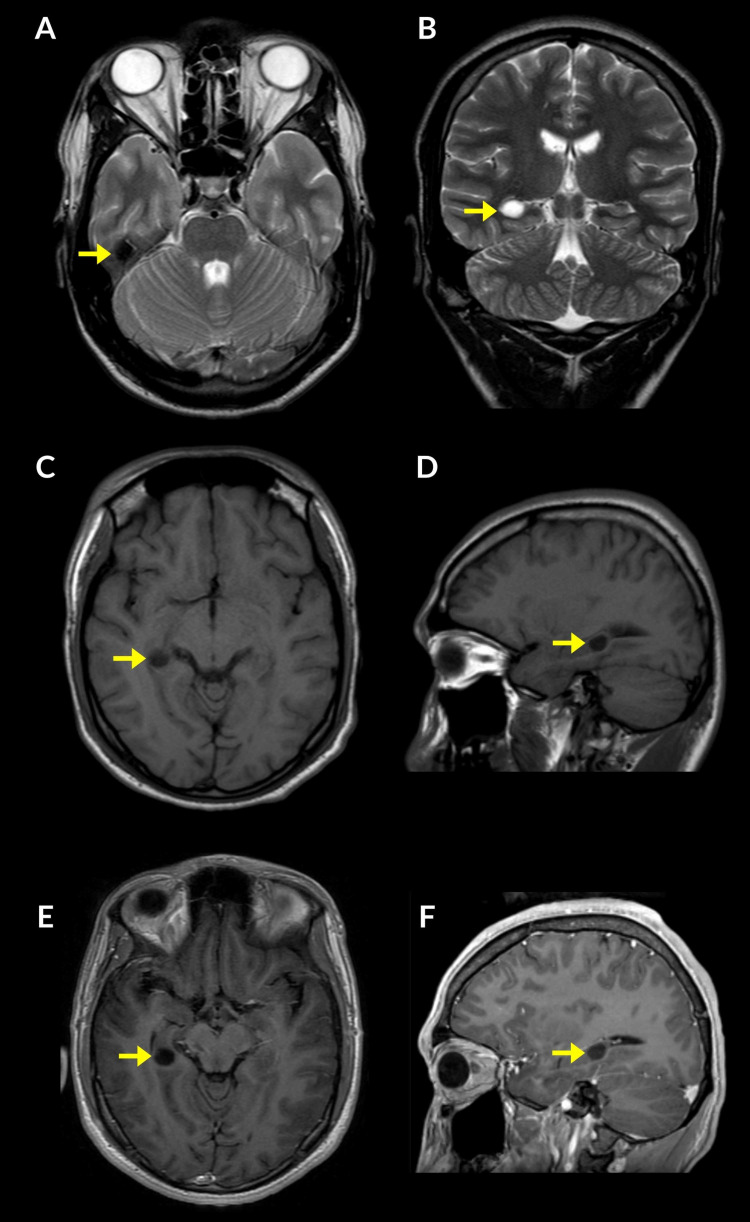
Brain magnetic resonance imaging (MRI) scan in a patient with DNET. Axial (A) and coronal (B) T2-weighted; pre-contrast axial (C) and sagittal (D) T1-weighted; and post-contrast axial (E) and sagittal (F) T1-weighted images showing the presence of an oval-shaped well-defined lesion in the body of the right hippocampus, which measures 10 x 8 x 10 mm in diameter (yellow arrows).
